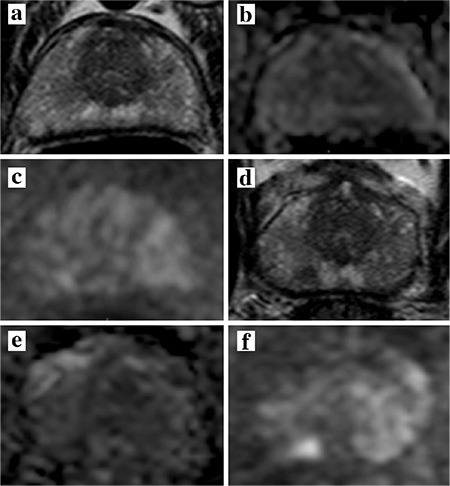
Fourty six-year old man with a serum PSA= 6.79 ng/mL with Gleason 3+3 prostate cancer diagnosis. Baseline mpMRI consisted of T2W MRI (a) ADC map (b) b1500 DW MRI (c) shows no lesion at the level of right apical portion of the prostate. Two year follow up axial T2W MRI (d) shows a lesion in the right apical peripheral zone, which is also positive on ADC map (e) and b1500 DW MRI (f) (serum PSA at 2 year follow up= 9.25 ng/mL). TRUS/MRI fusion biopsy revealed Gleason 4+3 with this lesion and patient became a radical prostatectomy candidate.
